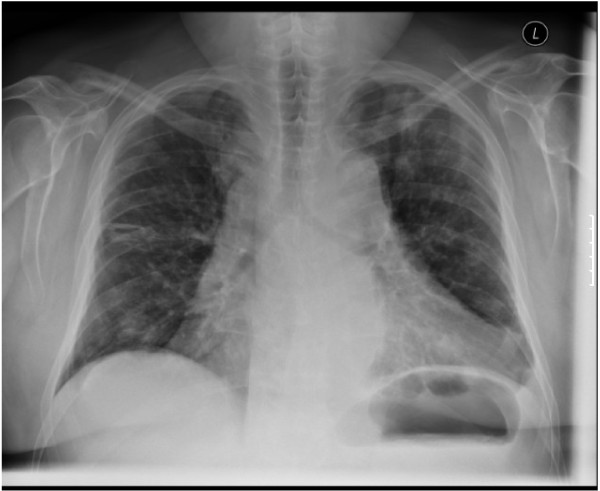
Chest radiograph following removal of chest drain. Complete re-expansion of the left lung as seen following removal of left-sided chest drain for spontaneous pneumothorax secondary to response to treatment with crizotinib.
